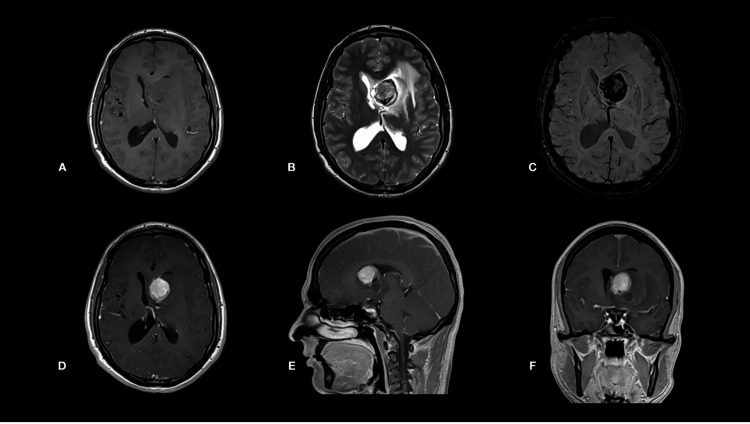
Preoperative brain MRI scan revealed (A) axial T1- and (B) T2-WI scans showing the intermediate signal intensity subependymal mass with dark T2 signal foci and cystic region. There is a significant mass blooming effect in the SDI-WI scan (C), indicating hemorrhages. C. Axial, sagittal, and coronal T1-WI scans (D-F) following intravenous contrast administration revealed heterogeneous enhancement of the left lateral ventricular tumor.
